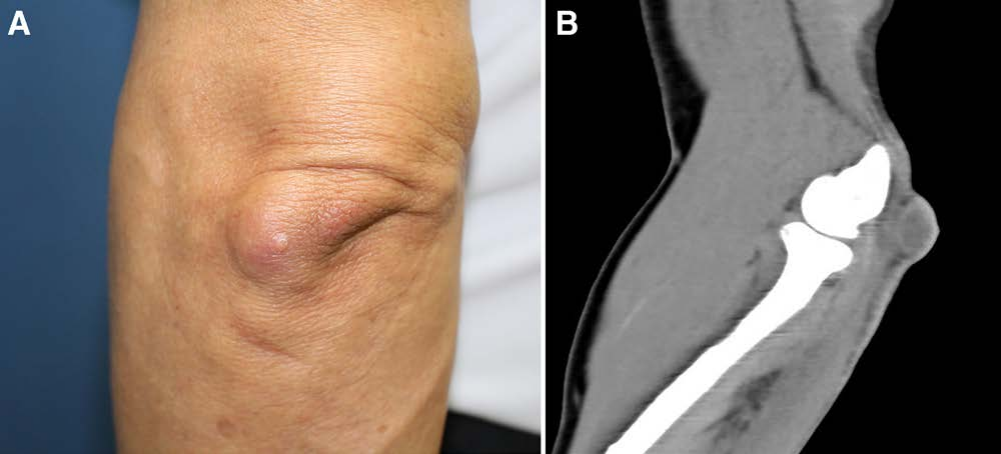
(A) Preoperative clinical photo. (B) The sagittal view of preoperative non-contrast computed tomographic image showing a subcutaneous cystic lesion at the posterior aspect of the radiocapitellar joint without invasion of deep structures.
